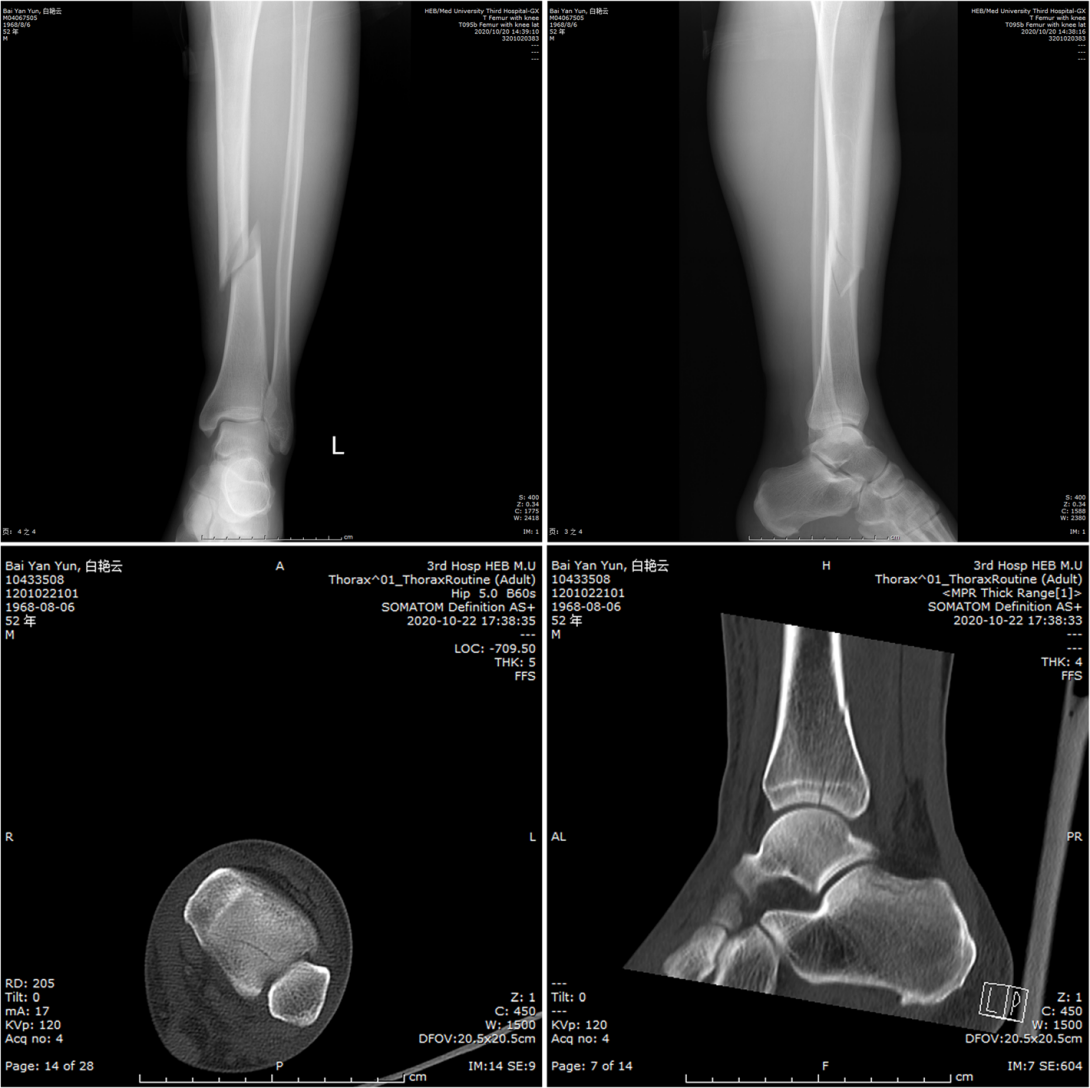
These radiological images show a non-displaced PMF associated with a TSF. a Anteroposterior and lateral tibia radiographs (including the ankle joint). b CT scans of the ankle joint (horizontal and sagittal views). The red arrow indicates the line of the PMF
